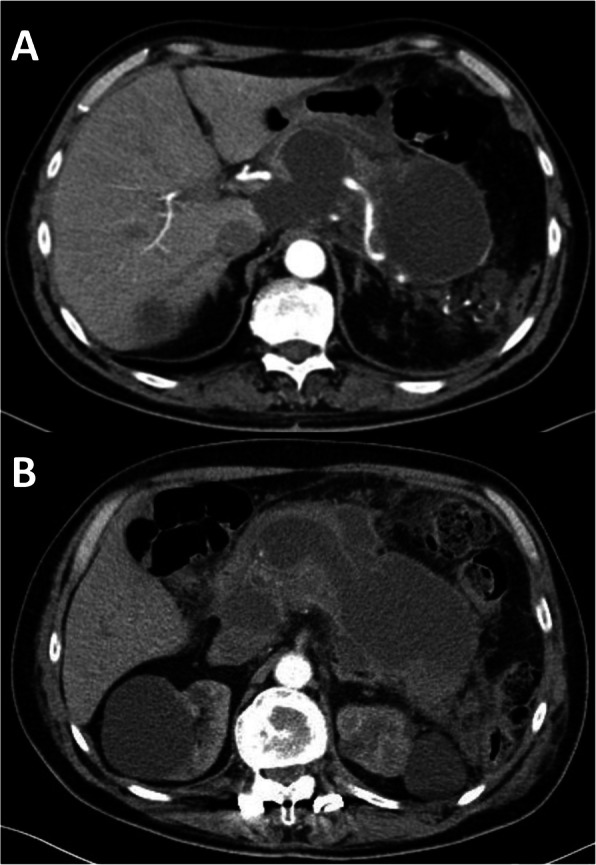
Case 1: Preoperative CT scan. a, b CT scan of the abdomen and pelvis revealing a heterogeneous 85 × 68 × 60-mm pancreatic necrotic collection
